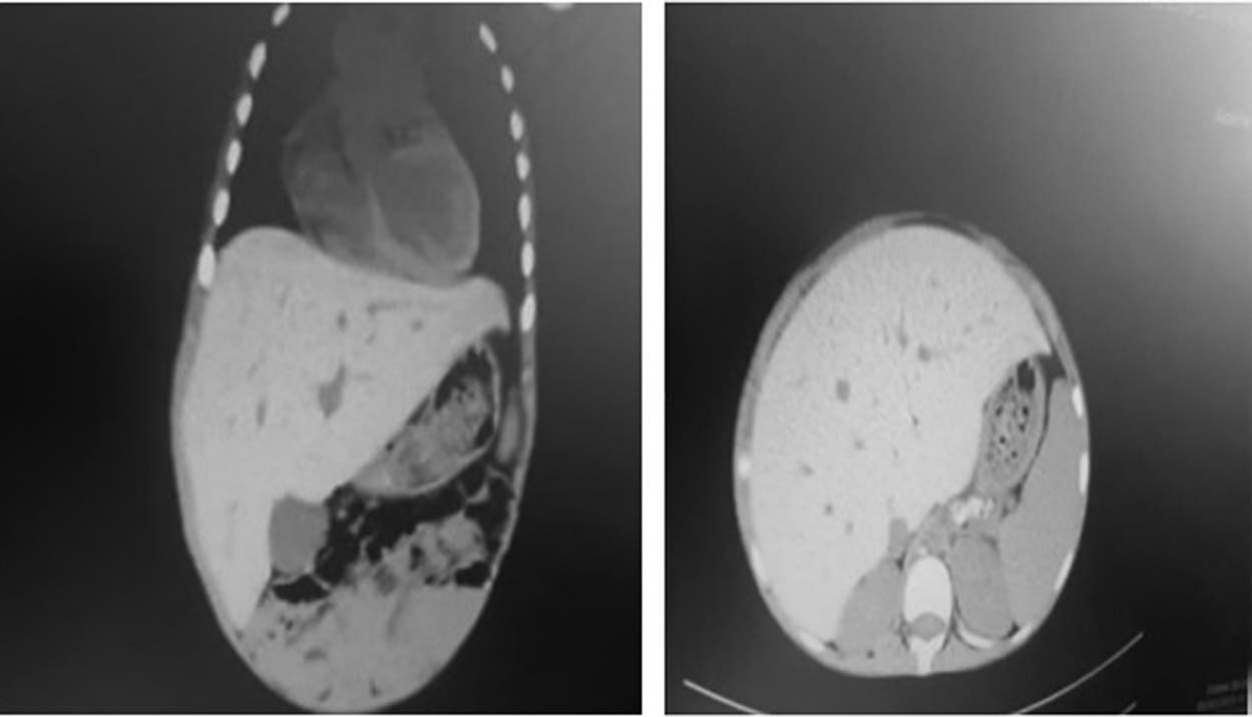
Computed tomography scan showed hepatomegaly and splenomegaly.
